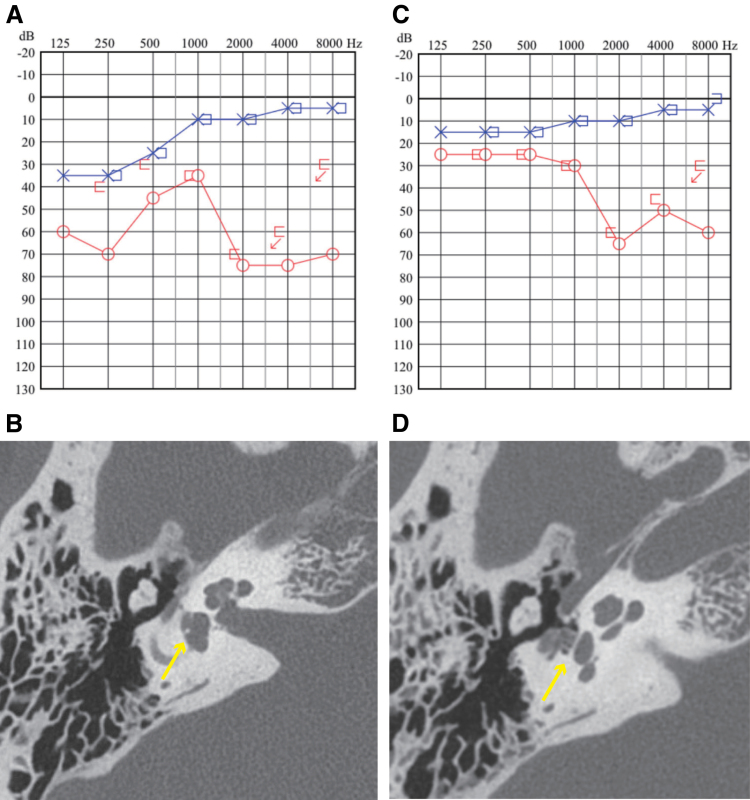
Preoperative and postoperative CT images and audiograms of Case 1. (A) Preoperative PTA. (B) Preoperative axial CT images of the temporal bone. The stapes footplate was completely invaginated into the vestibule (yellow arrow). (C) Postoperative PTA. (D) Postoperative axial CT images of the temporal bone. The stapes footplate was successfully repositioned in its anatomical place (yellow arrow). The arrow points to the cartilage placed on the oval window. The incus columella (arrowhead) was placed on the cartilage (arrow). The star indicates elevated TM for all figures. CT = computed tomography, PTA = pure-tone audiometry, TM = tympanic membrane.
